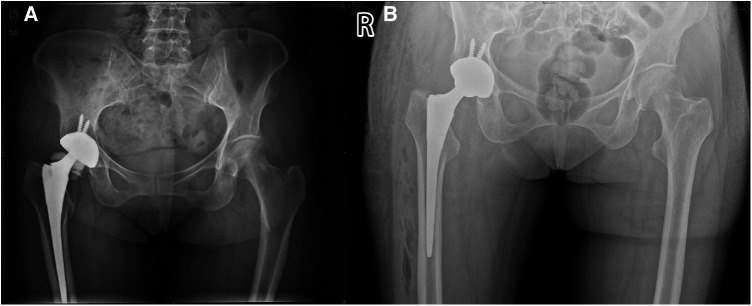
Pelvic and hip x-rays in case 1. (A) Preoperative anteroposterior radiographs of both hips. (B) Postoperative bedside DR anteroposterior radiographs of both hips.
